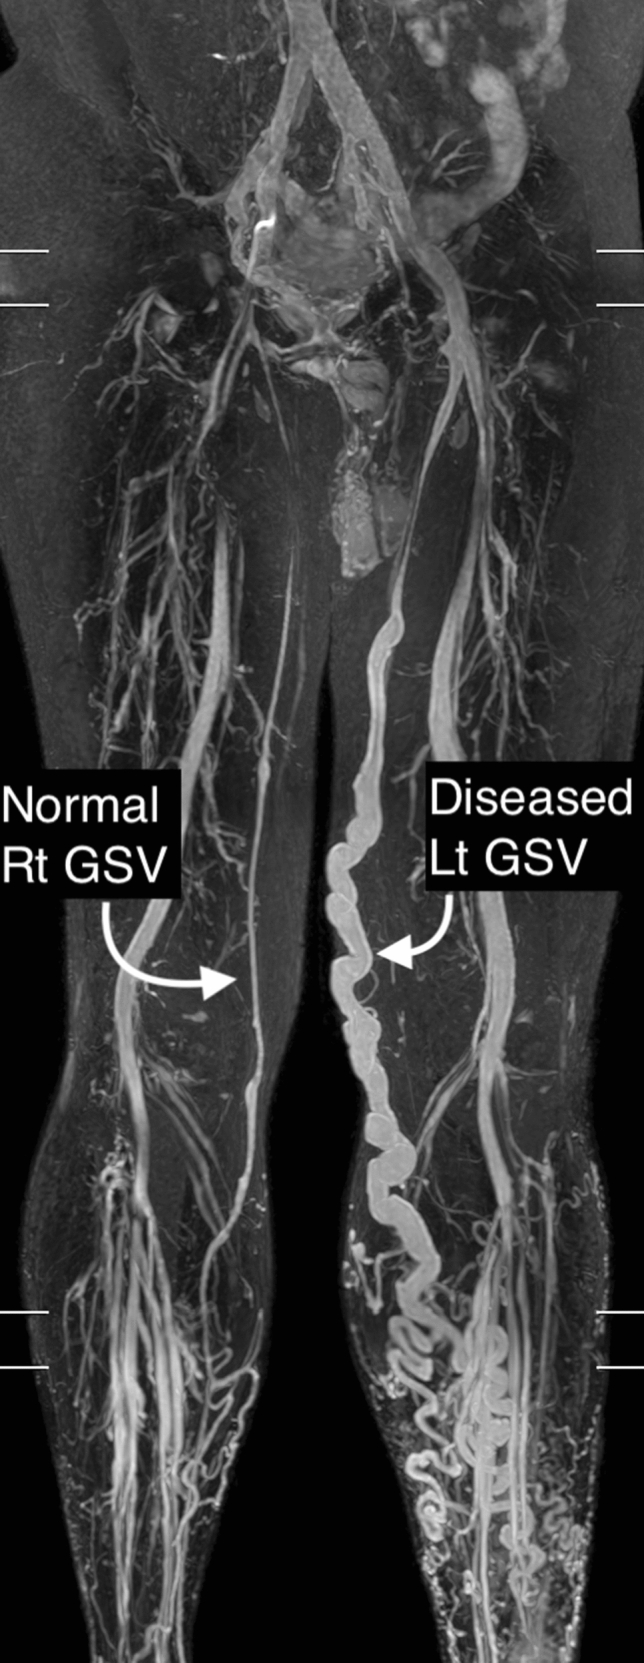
TRANCE-MRI image of a 68-year-old patient with venous reflux in the great saphenous vein of the left limb.
